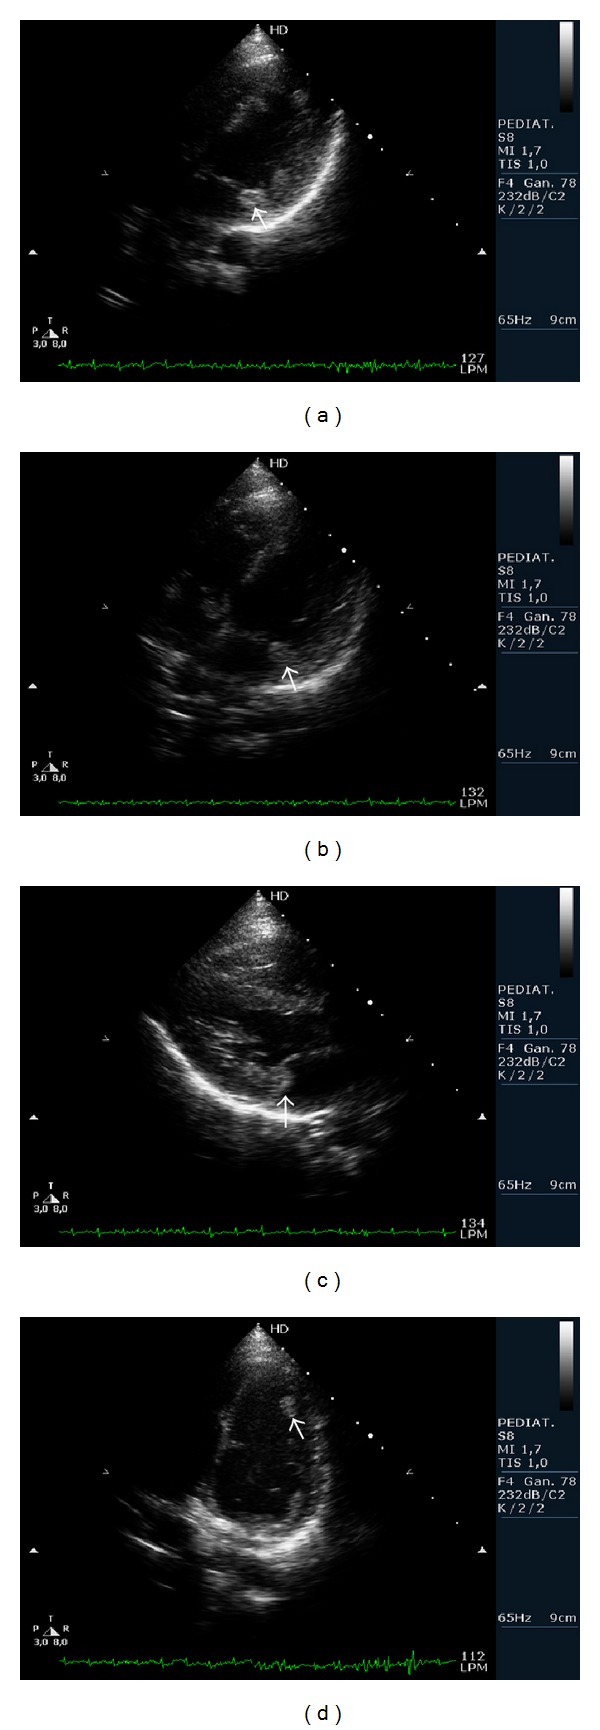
Royalty rhabdomyomas after 28-month followup. Note the disappearance of intramural rhabdomyomas and persistence of rhabdomyoma mainly located in the anterior leaflet of the mitral valve. (a) Four-chamber view with intracavitary mass in mitral valve (arrow). Disappearance of intraparietal mass in lateral wall of left atrium viewed in Figure 1(b). (b) Four-chamber doppler-colour view of intracavitary mass in tricuspid valve smaller than before, one intracavitary mass in mitral valve and disappearance of intraparietal mass in lateral wall of left atrium (arrow). (c) Longitudinal long axis of left ventricule with intracavitary mass on mitral valve (arrow). (d) Four-chamber view of intraparietal tumour in left ventricule apex smaller than before (arrow).
